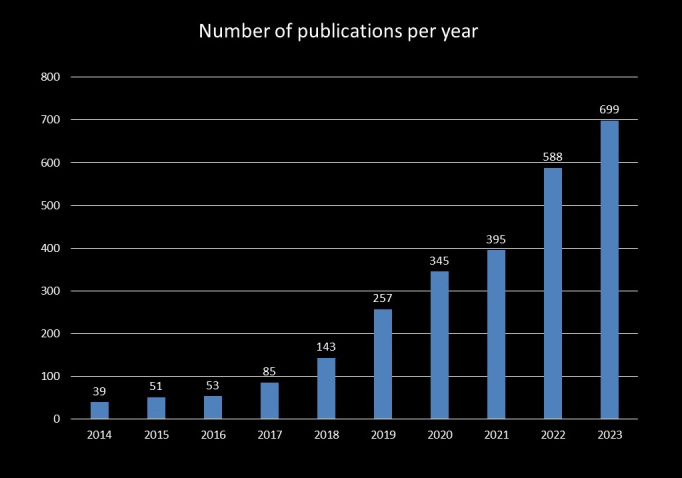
Annual Number of Publications on AI in Breast Ultrasound This figure shows the annual number of publications on Artificial intelligence (AI) in breast ultrasound from 2014 to 2023. The data, extracted from PubMed using a specific query, indicates a steady number of publications from 2014 to 2016, followed by a significant increase starting in 2017. Publications exceeded 100 annually since 2018, surpassing 300 in 2020, and reaching 699 in 2023.
